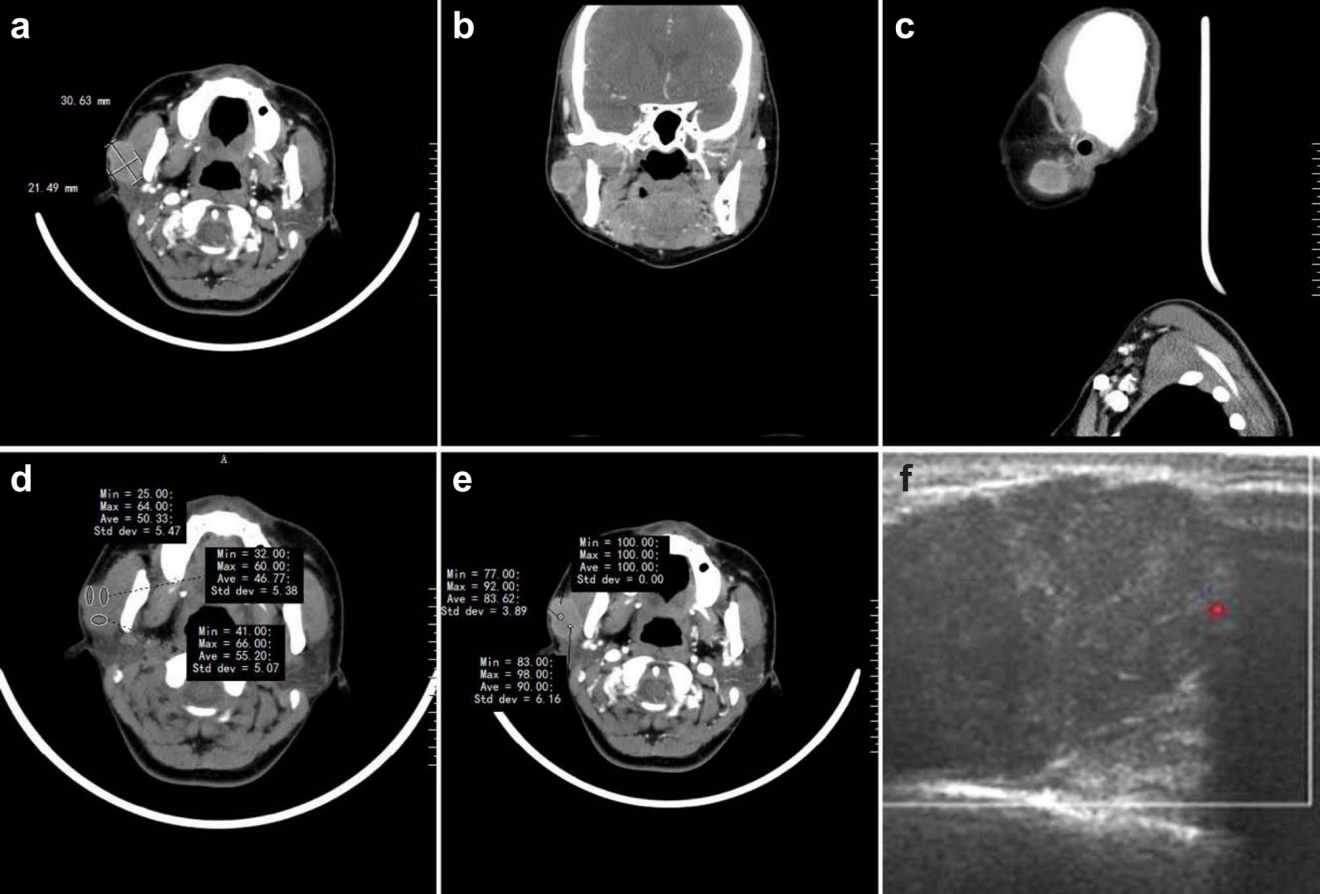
Color Doppler ultrasound and computed tomography (CT) imaging findings of typical SCSG. In CT, the lesions appeared oval or lobulated, with clear boundaries, regular edges, and uneven density. (a) Horizontal plane; (b) coronal plane; (c) sagittal plane. (d) Average Hounsfield unit (HU) was 42 (41.69 ± 16.5) with plain scanning, and (e) 76 (76.1 ± 24.7) with enhanced scanning. (f) Typical ultrasonic characteristics.
